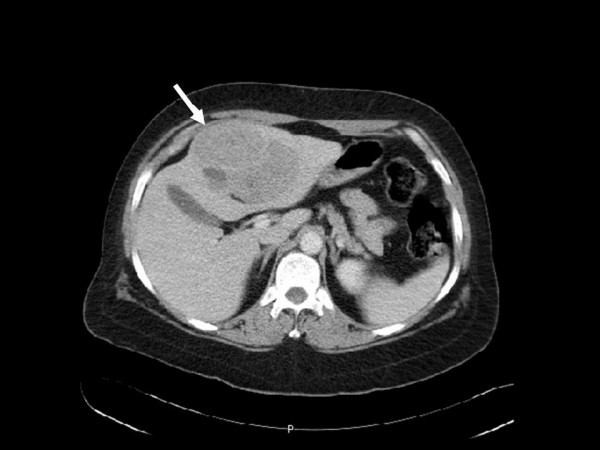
CT image showing the metastatic lesion in segments 2, 3 and 4 of the left hepatic lobe.
